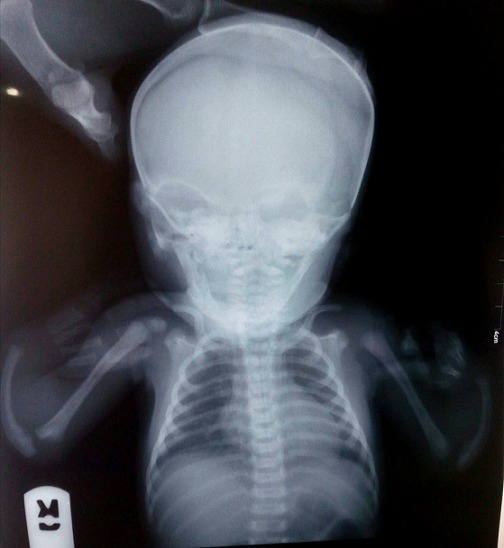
Radiograph of both upperlimbs showing absence of radius bilaterally and ulnar deviation of the hand
